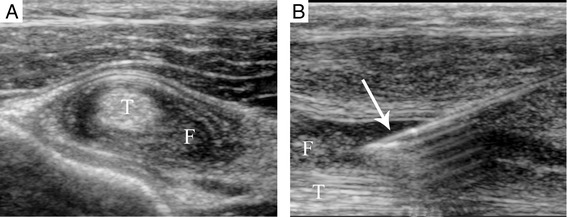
6 years 8 months old male referred for injection of biceps brachii tendon bilaterally. a Ultrasound showed echogenic peritendinous fluid and thickening of the sheath around the tendon (T). b The fluid was aspirated with a 20-gauge angiocath™ (*arrow*) and sent for culture
